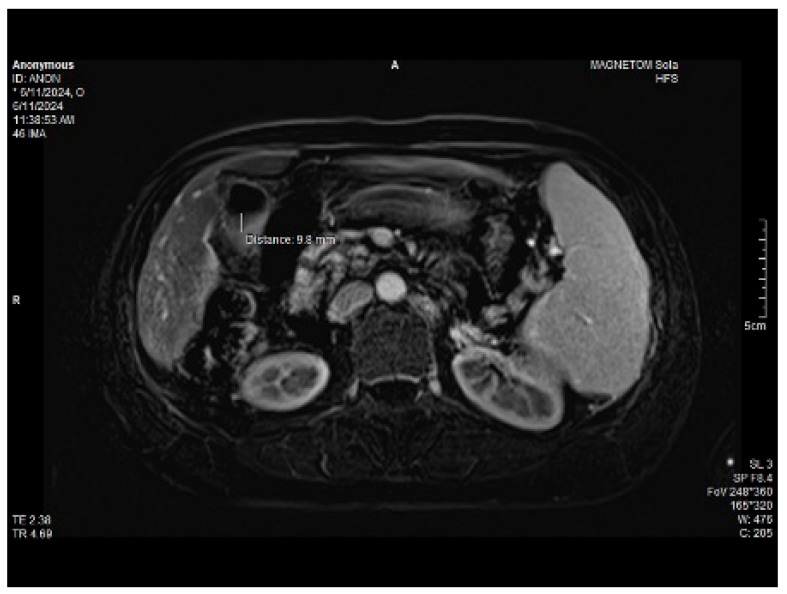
Massive thickening of the gallbladder wall, MRI features.
